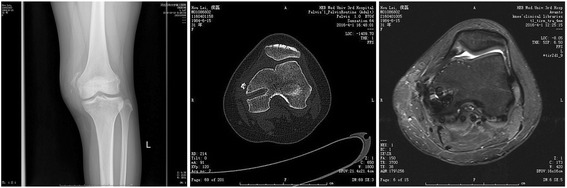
Post-operative heterotopic ossification at the femoral tunnel can be diagnosed by X-ray, CT scan and MRI scan, respectively
